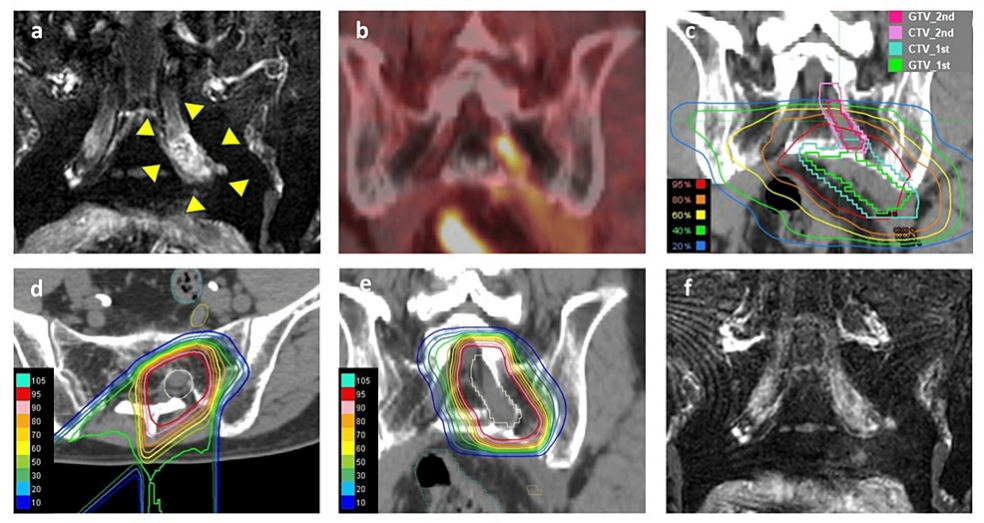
MRI and FDG PET-CT images pre and post-proton beam re-irradiation for localized PNS. Contrast-enhanced MRI (a: coronal), FDG PET-CT (b: coronal), and the relationship of the PNS lesion and first PBT dose. The first gross tumor volume (GTV) is enclosed by the green line. The first clinical target volume (CTV) is enclosed by the blue line. The second GTV is enclosed by a magenta line. The second CTV is enclosed by a pink line (c: coronal). Dose distribution for the proton beam re-irradiation (d: axial, e: coronal). Post-treatment MRI (f: coronal) showed a complete response one year after proton beam re-irradiation. In the images, the recurrent lesion is indicated by a yellow triangle. The dose lines are color-coded as follows: Red line: 95% isodose line, Yellow line: 75% isodose line, Green line: 50% isodose line, Light blue line: 25% isodose line. FDG PET-CT: 18 F-fluorodeoxyglucose Positron Emission Tomography–Computed Tomography
